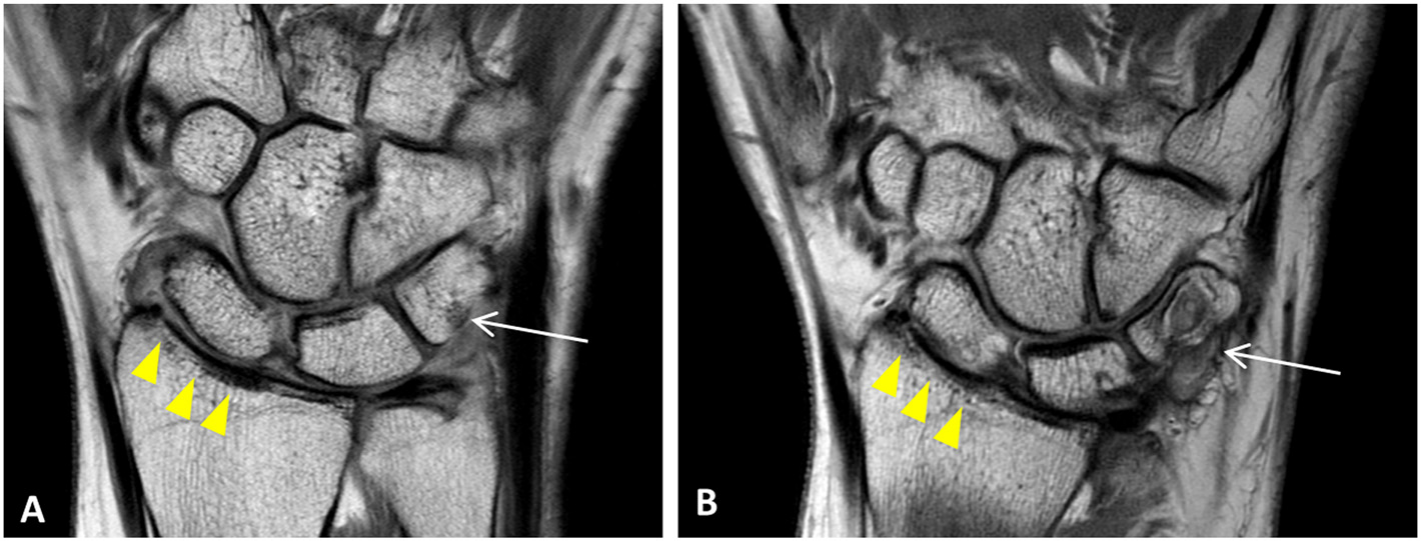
Coronal proton density MRI wrist scans. A) Baseline MRI scan (2009) shows cartilage thinning at the radiolunate (score = 2 ) and radioscaphoid joints (score = 1) (arrowheads) B) At 3 years (2012), the cartilage space narrowing has progressed; radiolunate score = 3 and radioscaphoid joint score = 3 (arrowheads). A new erosion (white arrow) is also now seen to involve the triquetrum at the site of bone oedema at baseline.
